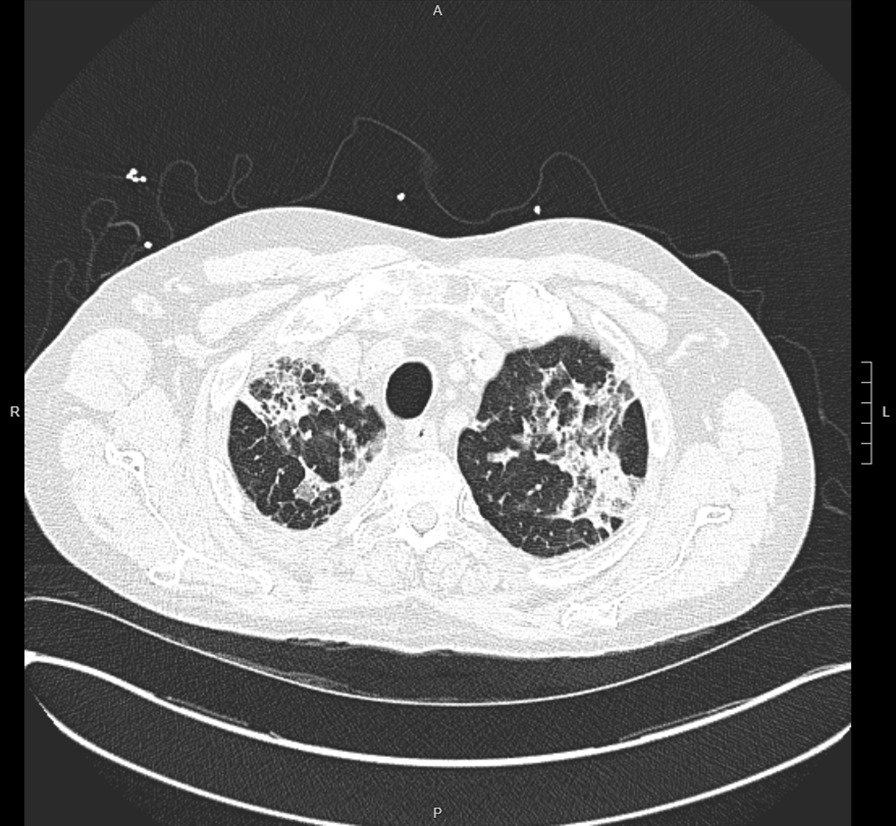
CT high resolution—chest
